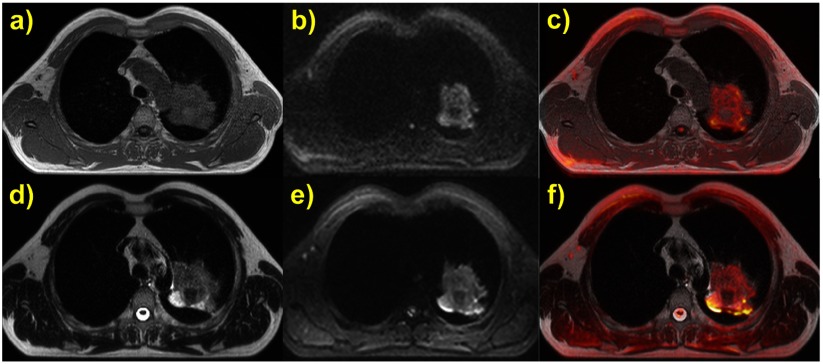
MR images of a NSCLC patient acquired in ABC breath holds with the same inhalational threshold: (*a*) T1-w 3D VIBE (slice location 57.56 mm, thickness 3 mm); (*b*) DW-EPI2 with *b* = 750 s mm^−2^ (slice location 57.17 mm, thickness 6 mm); (*c*) overlay of (*a*) and (*b*) in OsiriX. (*d*) T2-w HASTE (slice location 58.67 mm, thickness 3 mm); (*e*) DW-EPI1 with *b* = 200 s mm^−2^ (slice location 58.67 mm, thickness 5 mm) and (*f*) their overlay in OsiriX. All original images were individually windowed.
